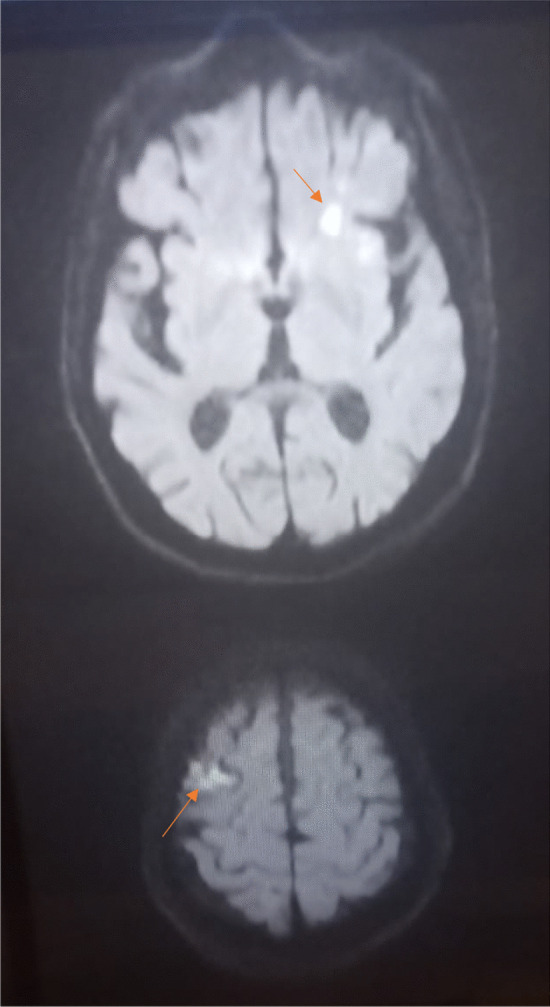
Brain MRI showing a right frontal lesion evoking a cerebral abscess and a left parietal lesion evoking an ischemic stroke at the subacute stage
